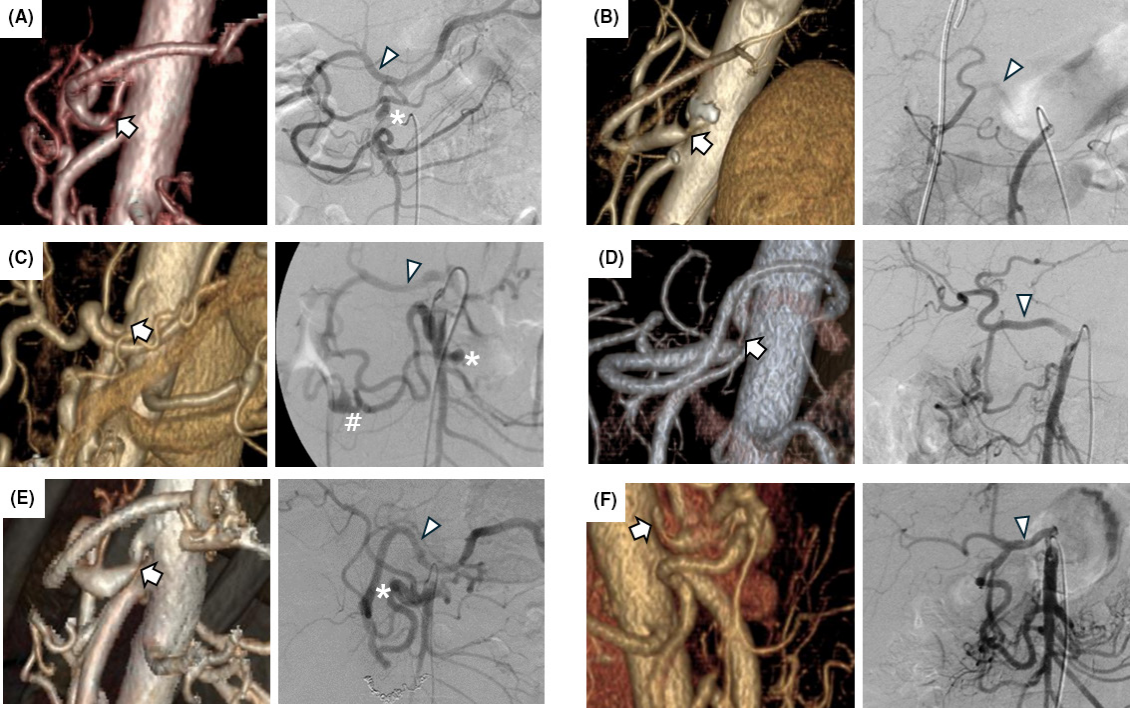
Characteristics of median arcuate ligament syndrome. Left panels, 3D CT‐angiography showing the celiac artery stenosis or obstruction (arrow). Right panels, angiography from the superior mesenteric artery. Retrograde contrast material was observed in the common hepatic arteries in all cases (arrowheads). (A) Case 1: Reflux to the level of the splenic artery. A pancreaticoduodenal artery aneurysm is visible (asterisk). (B) Case 2: Stents have been inserted for biliary obstruction owing to carcinoma of the papilla of Vater. (C) Case 3: A ruptured aneurysm is visible (indicated by #). (D) Case 4. (E) Case 5: After coiling for hemostasis. Reflux to the level of the splenic artery is visible, with a pancreaticoduodenal artery aneurysm (asterisk); (F) Case 6: Donor patient's findings prior to living donor liver transplantation. 3D, three‐dimensional; CT, computed tomography.
